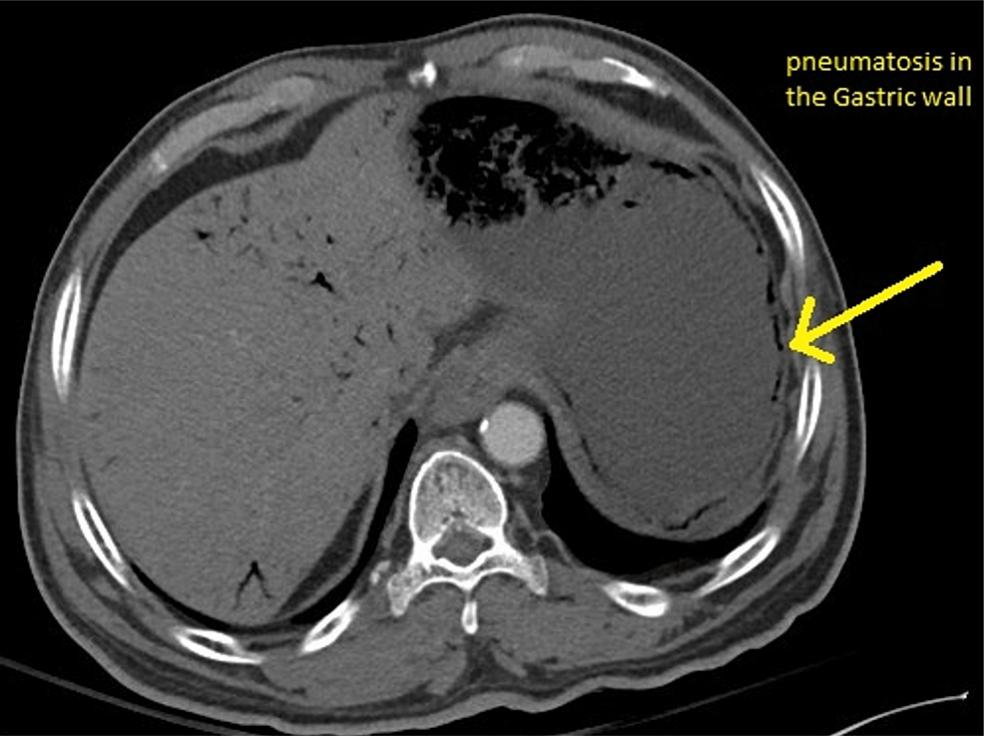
Abdominal CT scan showing gastric wall pneumatosis (yellow arrow)
